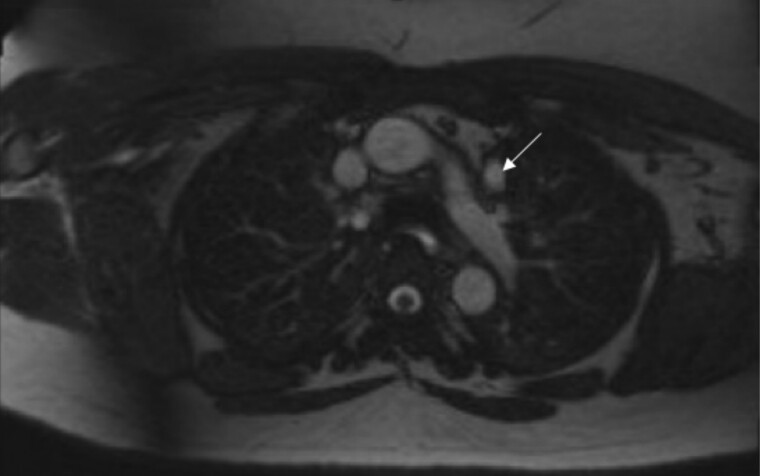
Axial magnetic resonance imaging of the chest shows a persistent left-sided superior vena cava (white arrow). The normal right-sided vena cava is also present.
